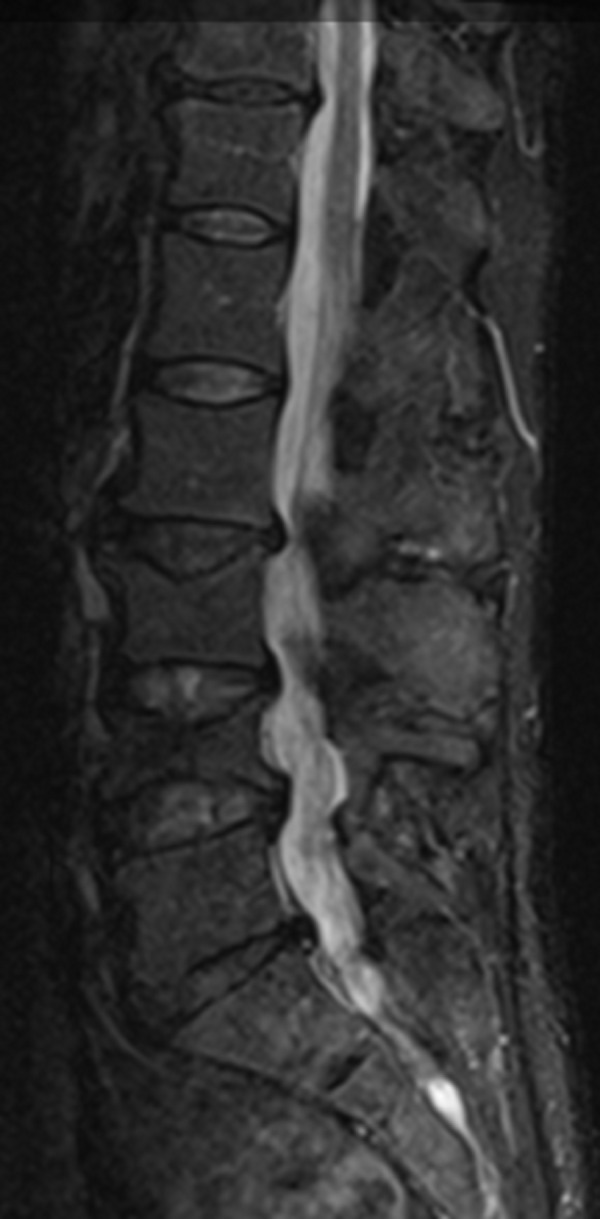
(Case 2): Preoperative sagittal MRI of the lumbar spine
